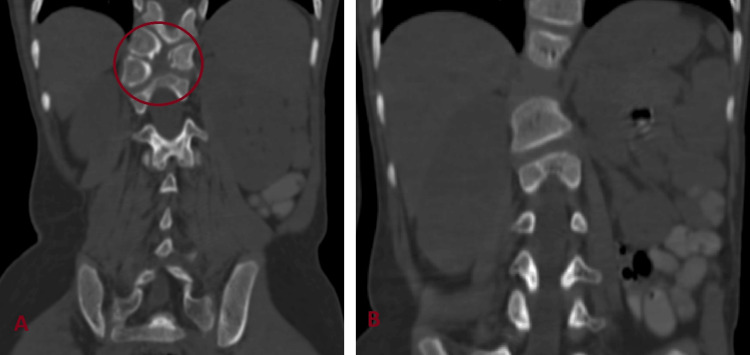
CT coronal sections in bone window showing (A) butterfly vertebra and hemivertebra at D11 and D12 level respectively (red circle) and (B) dextroscoliosis CT - computed tomography
